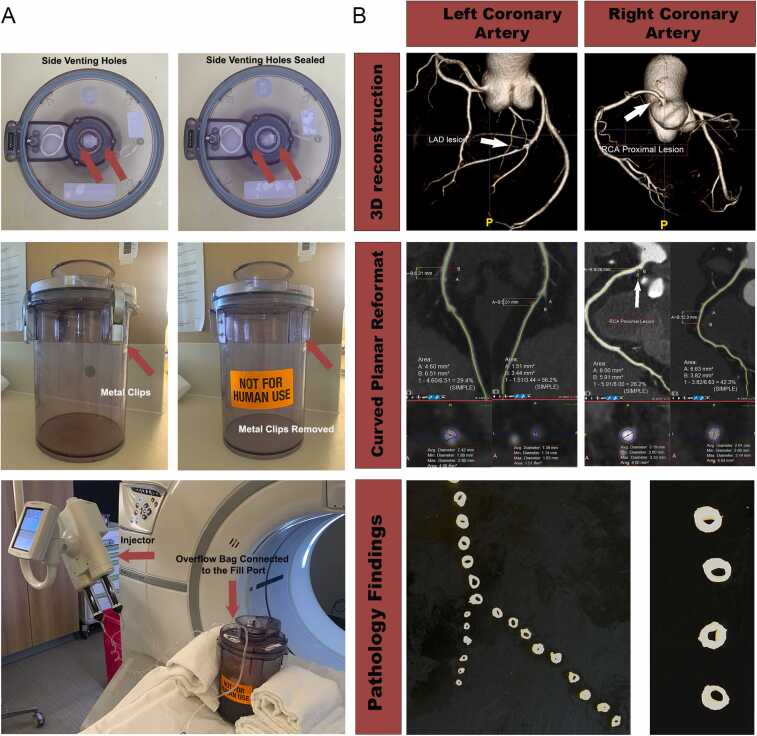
(A)Technical modifications and procedural details of coronary CTA of a donor heart in Paragonix SherpaPak. The side venting ports had to be sealed and metal clips removed from the commercially available device (red arrows). The contrast was injected through an extension tubing attached to the central vent port on the lid of the inner container. (B) 3D reconstruction of the aortic root and coronary arteries demonstrating lesions in the proximal RCA and mid LAD. Quantitative analysis was performed using curved planar reformat technique. Cross sections of the coronary arteries confirming luminal narrowing. 3D, three-dimensional; CTA, computed tomography angiography; LAD, left anterior descending; RCA, right coronary artery.
